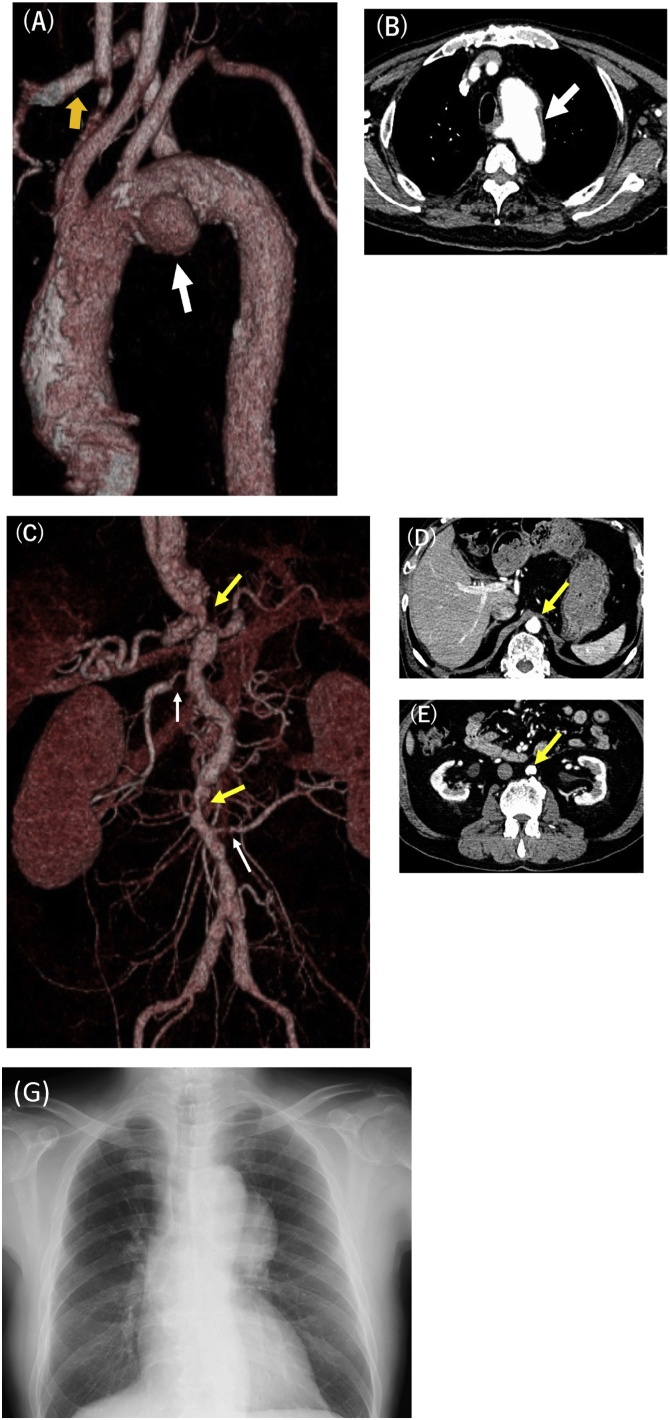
Preoperative computed tomography images. (A) Saccular type aortic arch aneurysm (solid arrow) and aberrant right subclavian artery (white arrow). (B) Shaggy aorta was acknowledged (white arrow). (C) Aortic stenosis at the level of the thoracoabdominal aorta and infra-renal abdominal aorta (gold arrows). Right renal artery had severe stenosis (white arrow). (D, E) Abdominal aorta was 19 mm just above celiac artery (CA), it become more stenosis at renal level (13 mm).
